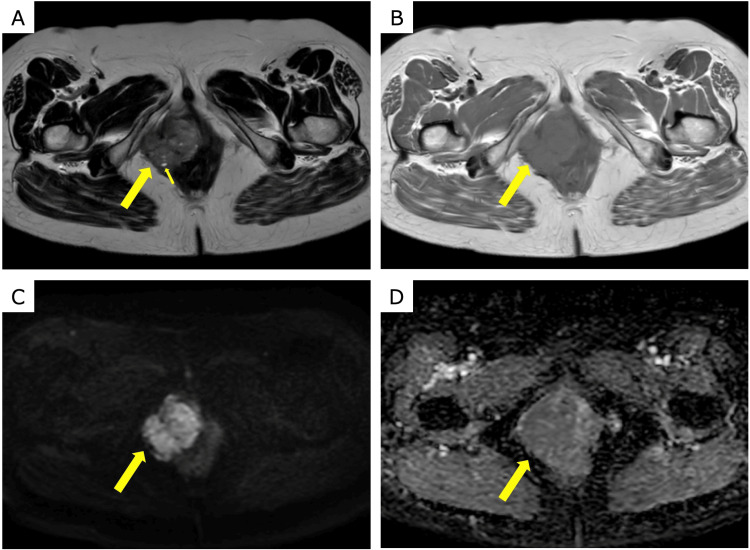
Pelvic MRI performed at our institution (A) T2-weighted image showing heterogeneous signal intensity, predominantly mildly hypointense with areas of mild hyperintensity, including small high-signal foci (small arrow) suggestive of cystic components. (B) Unenhanced T1-weighted image showing that the lesion is isointense to the adjacent muscle, with no fat or hemorrhagic components. (C, D) Diffusion-weighted image (C, b = 1000 s/mm²) shows high signal, whereas the apparent diffusion coefficient map (D) shows a value of 1.23 × 10⁻³ mm²/s, without restricted diffusion MRI: magnetic resonance imaging
